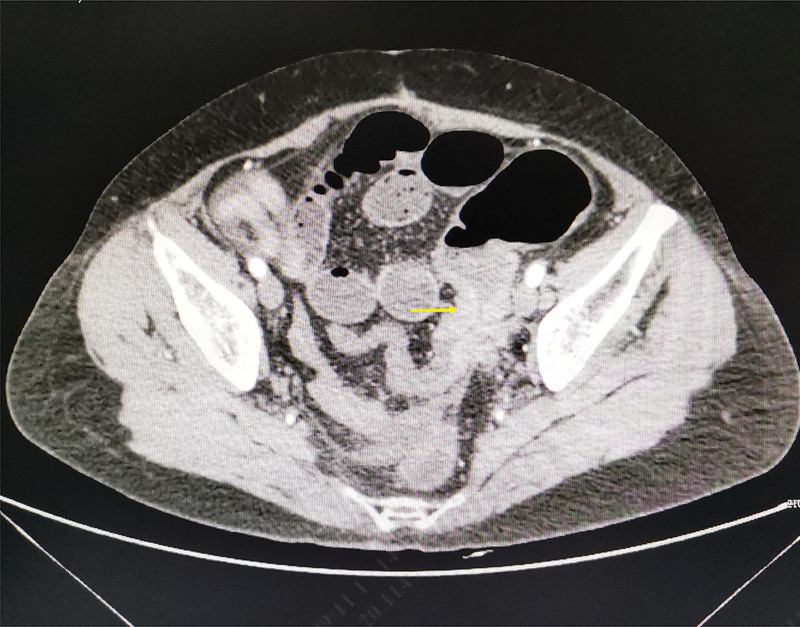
Contrast-enhanced CT image shows a mass, narrowing the lumen of the sigmoid colon. CT = computed tomography.
